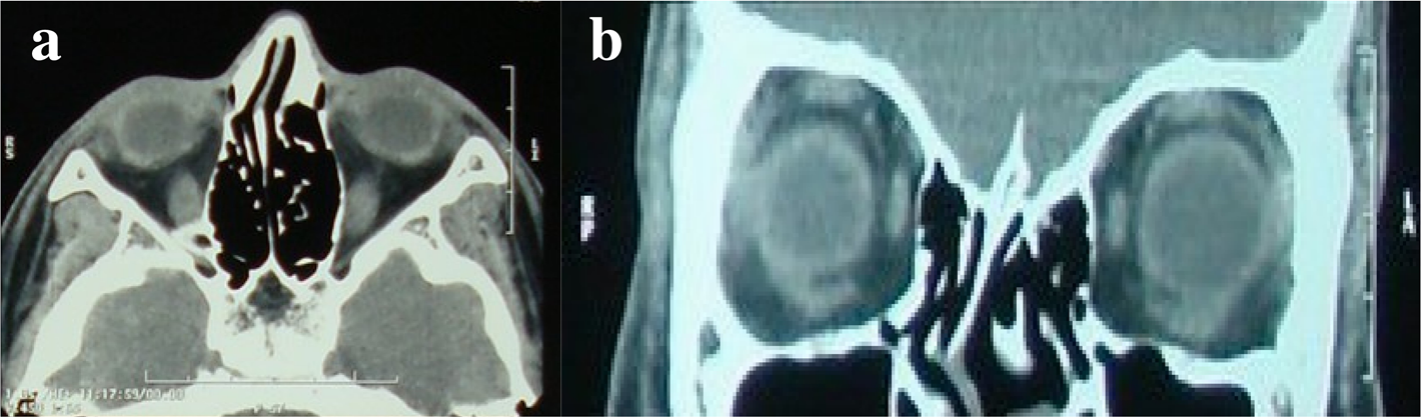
Postoperative imaging. Orbital CT scan reveals mild secondary hyperostosis in the previous location of the mass, with reasonable healing and no remnant of the original tumor (a: axial section, b: coronal section)
